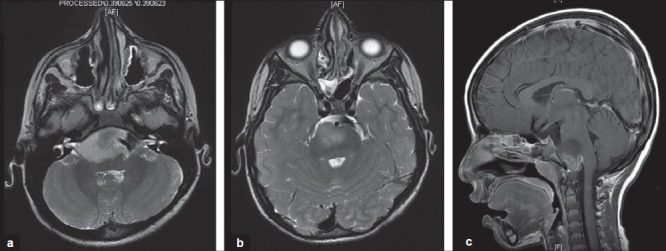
Three MRI of the patient under study showing lesion occupied most of the anterior pons and that was ventrally exophytic going down; hypodense on CT and hypo intense on MRI. The lesion surrounded completely incased a part of the vertebral junction and had component that enhanced with gal linear infusion. No part of these lesions was hypodense on CT and hypo intense on MRI.
